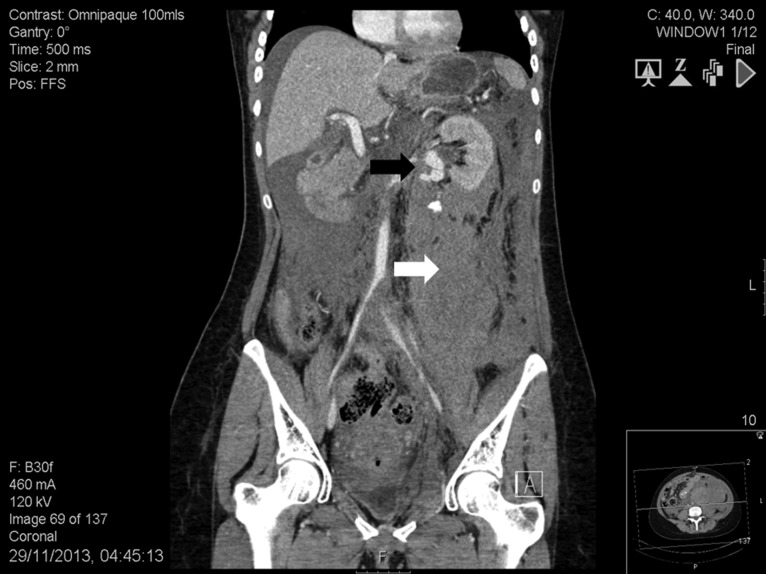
Abdomen contrast-enhanced computed tomography scan: massive retroperitoneal haematoma (white arrow) with active bleeding from a ruptured 2-cm left renal artery aneurysm (black arrow).
